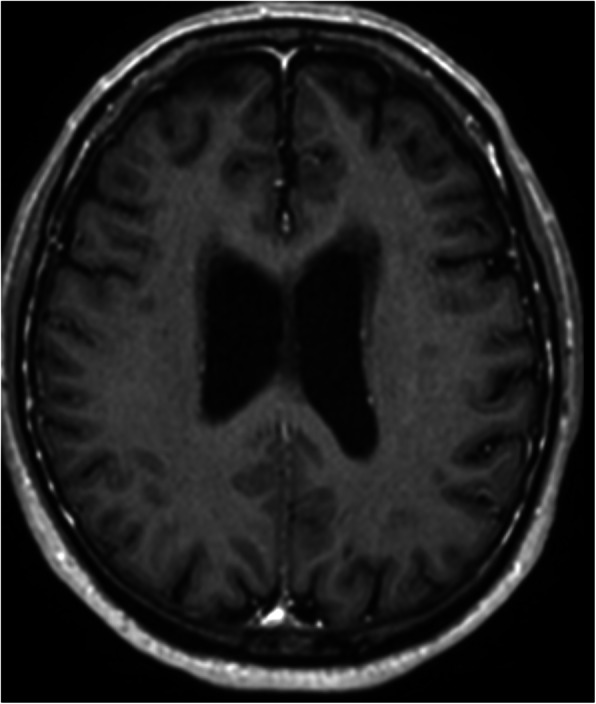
MRI image 3D T1 TFE + gadolinium demonstrating some periventricular white matter lesions and a mild cortical and subcortical atrophy. There is no abnormal meningeal enhancement
